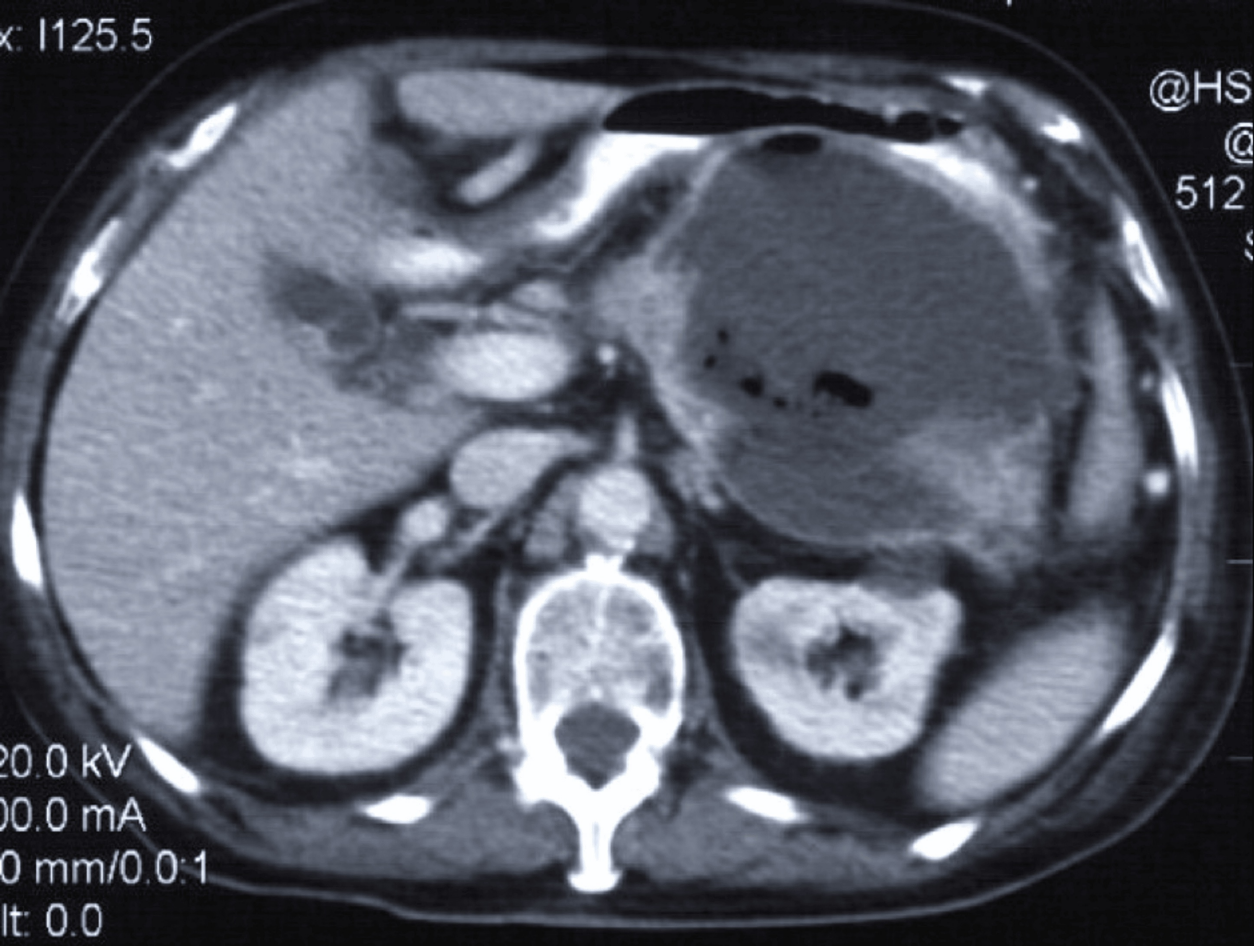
Enhanced computed tomography showing a well-circumscribed, thick-walled pancreatic pseudocyst compressing the gastric wall. Cyst wall enhancement and gas within the cyst suggest an infected pancreatic pseudocyst.
